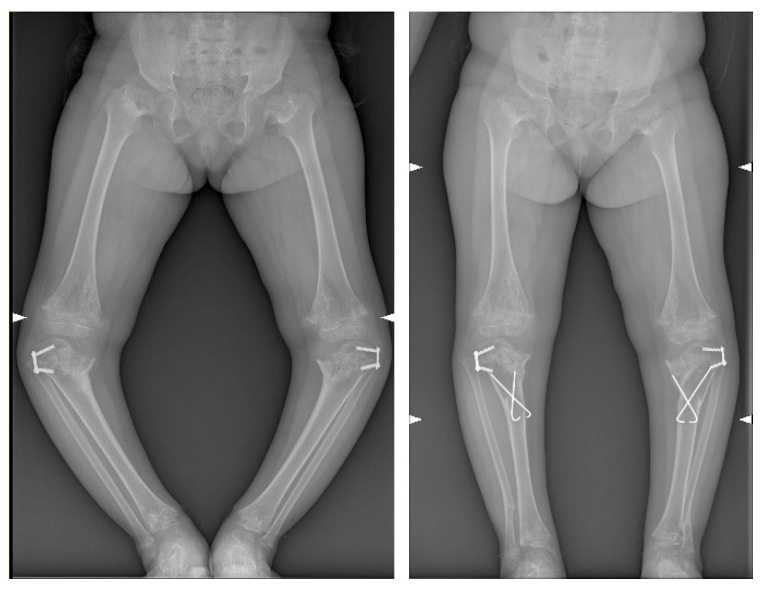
Preoperative and postoperative long-leg weight-bearing anteroposterior radiographs.
